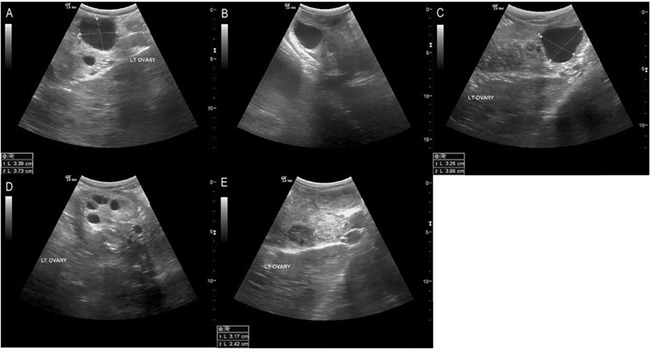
Ultrasound images of follicle progression through GnRH treatment. Representative ultrasound images of follicle progression at GnRH treatment through subsequent exams at 24, 36 and 48 hours post-administration, and CL formation. (A) Follicle on the day of GnRH treatment; (B) 24 hours post-treatment; (C) 36 hours post-treatment, note the shape change; (D) 48 hours post-treatment, the absence of the follicle indicative of ovulation. (E) CL evident following treatment.
